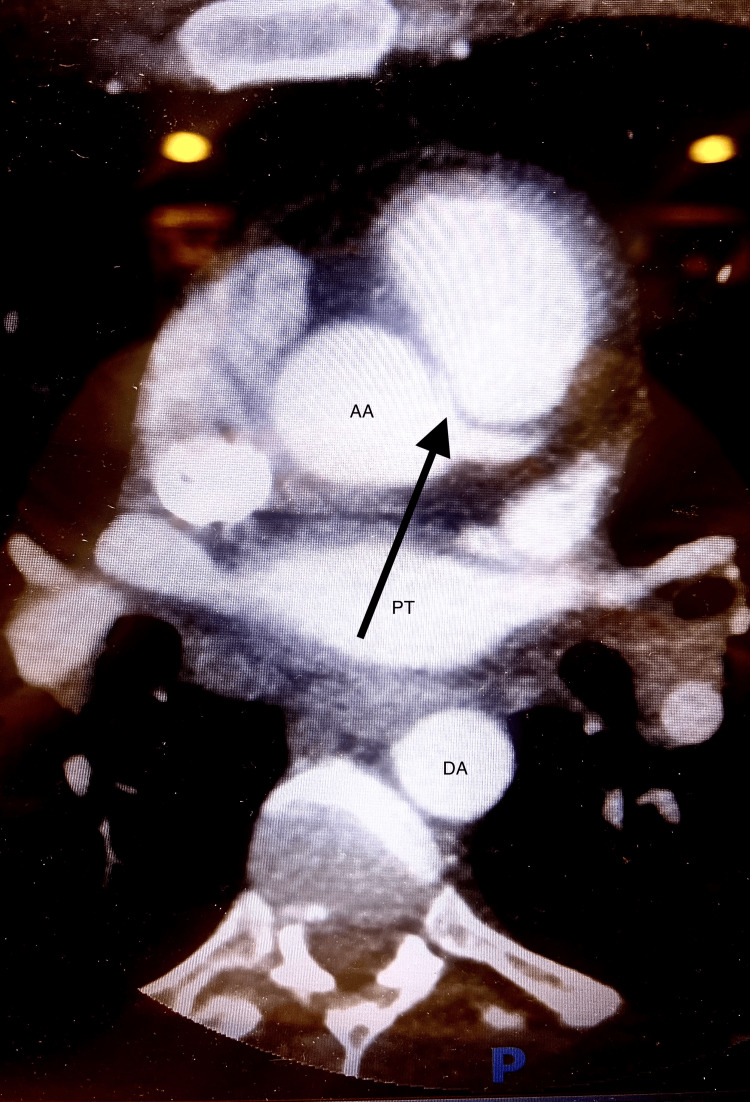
Coronary computed tomography angiography revealing an anomalous origin of the RCA originating from the left coronary cusp The arrow points to the anomalous origin of the RCA. AA, ascending aorta; DA, descending aorta; PT, pulmonary trunk; RCA, right coronary artery
